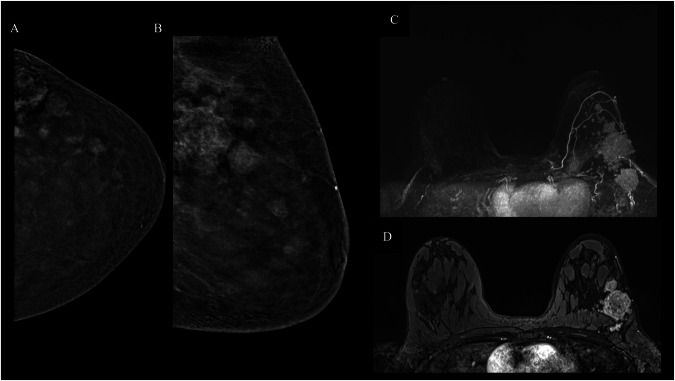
Posterior breast cancer in a patient with childhood brachial plexus palsy. The MLO view could not be optimally performed due to limited arm mobility. Recombined CEM images (A, B) show suspicious enhancement in the posterior breast. MRI maximum intensity projection (C) reveals an additional enhancing lesion in the axillary tail. Post-contrast non-subtracted T1-weighted MRI image (D) shows tumour extension with infiltration of the pectoralis muscle
